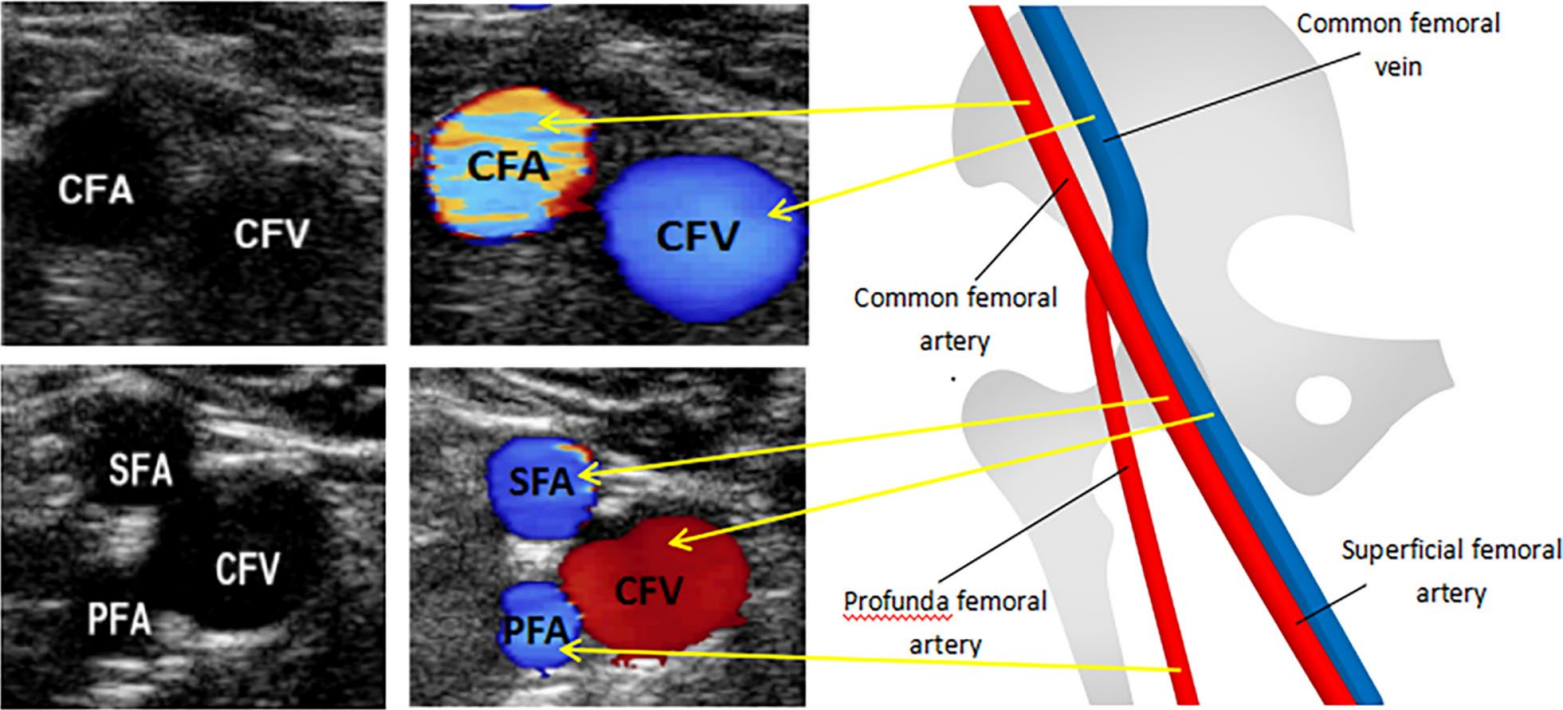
The images of femoral artery and vein were measured by ultrasound
